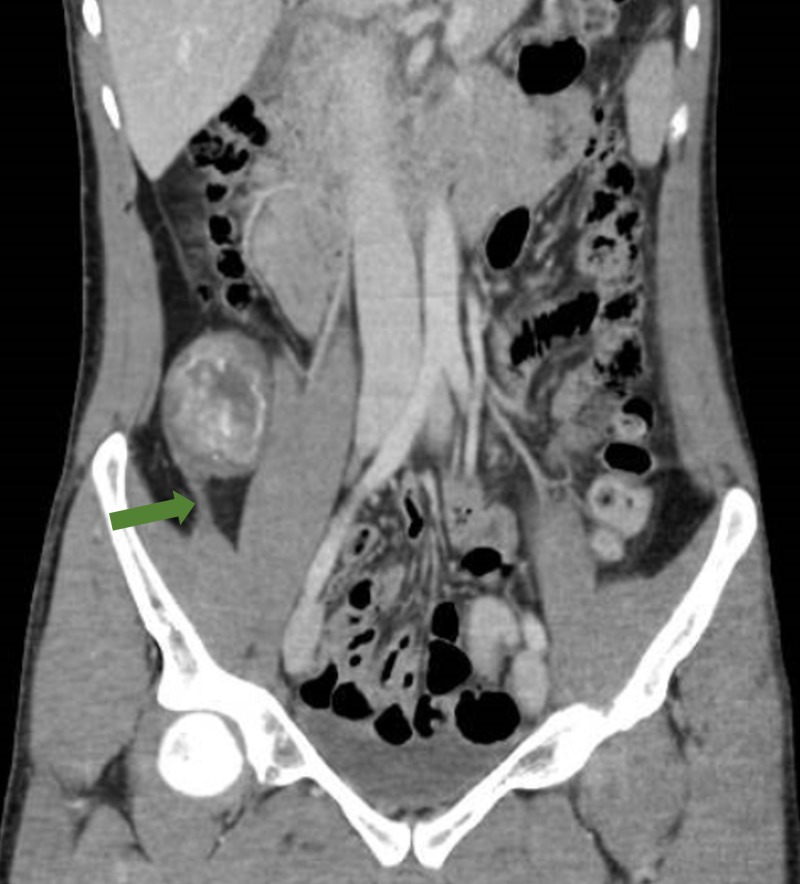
Computed tomography of the abdomen and pelvis in portal venous phase (coronal reconstruction). The inferior tail of the lesion (green arrow) is visible on this reconstruction.
